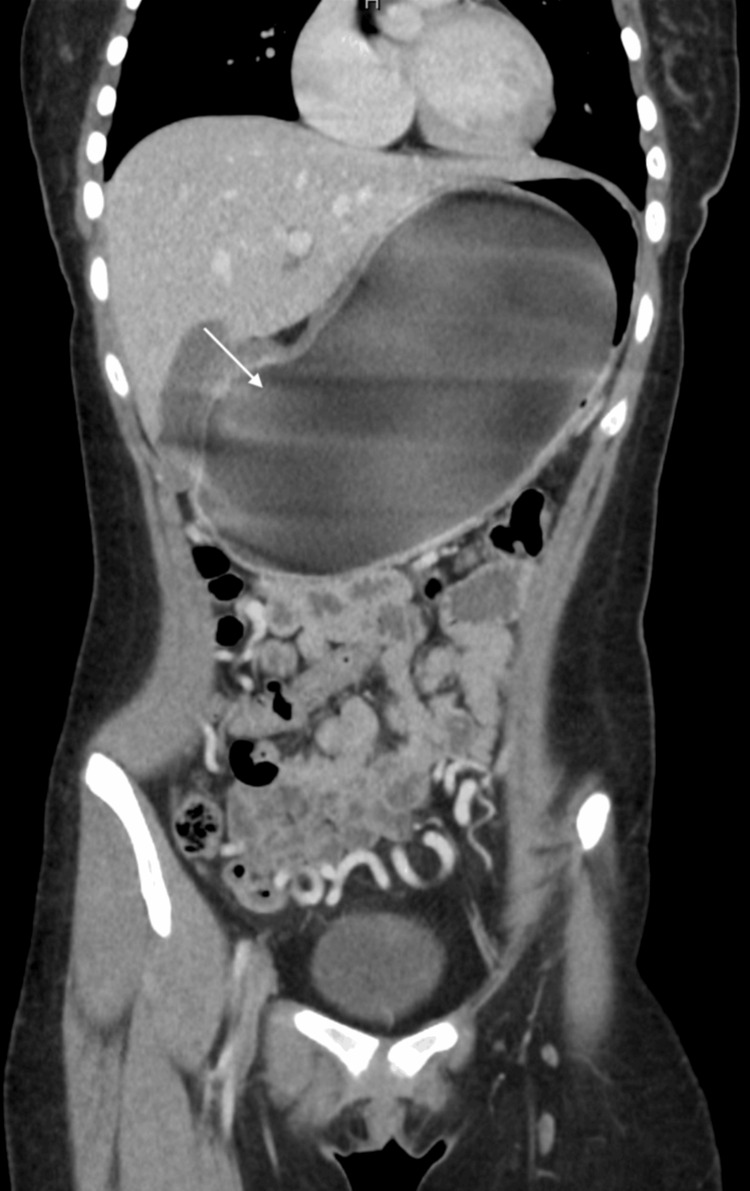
Coronal view of CT scan of patient’s abdomen with the intragastric balloon from referral hospital
